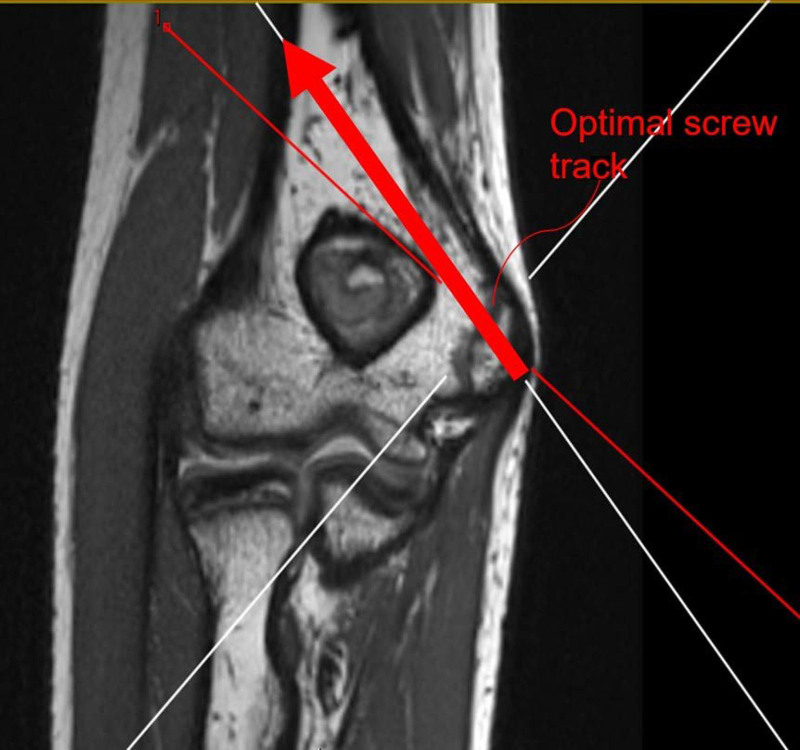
Coronal view of MRI displaying ideal implant track with entry near perpendicular to the medial epicondyle and cranial to the olecranon fossa. MRI = magnetic resonance imaging.
